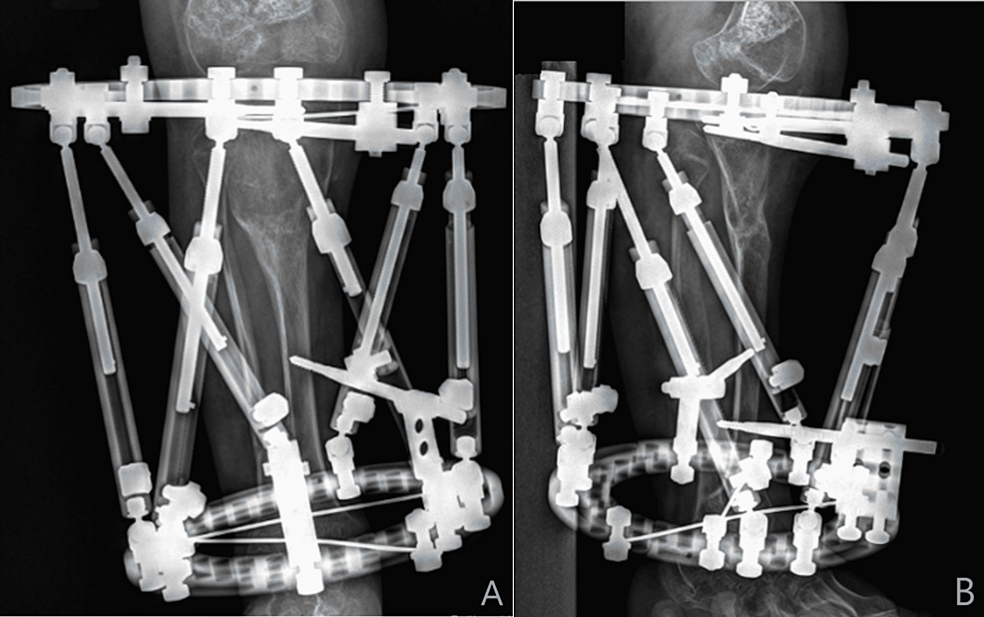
Radiography at five months postoperatively Anteroposterior (A) and lateral (B) radiographs of the leg five months post-operatively demonstrate a proximal healing tibial osteotomy site with callus and sclerosis.
